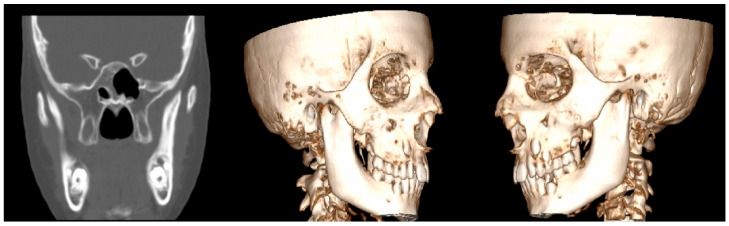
Coronal CT slice (left) and 3D reconstructed views (center and right) demonstrating bilateral elongation of the coronoid processes. Additionally, heterotopic bone formation is visible on the medial and inferior surfaces of the bilateral zygomatic arches, contributing to the patient’s limited mouth opening.
